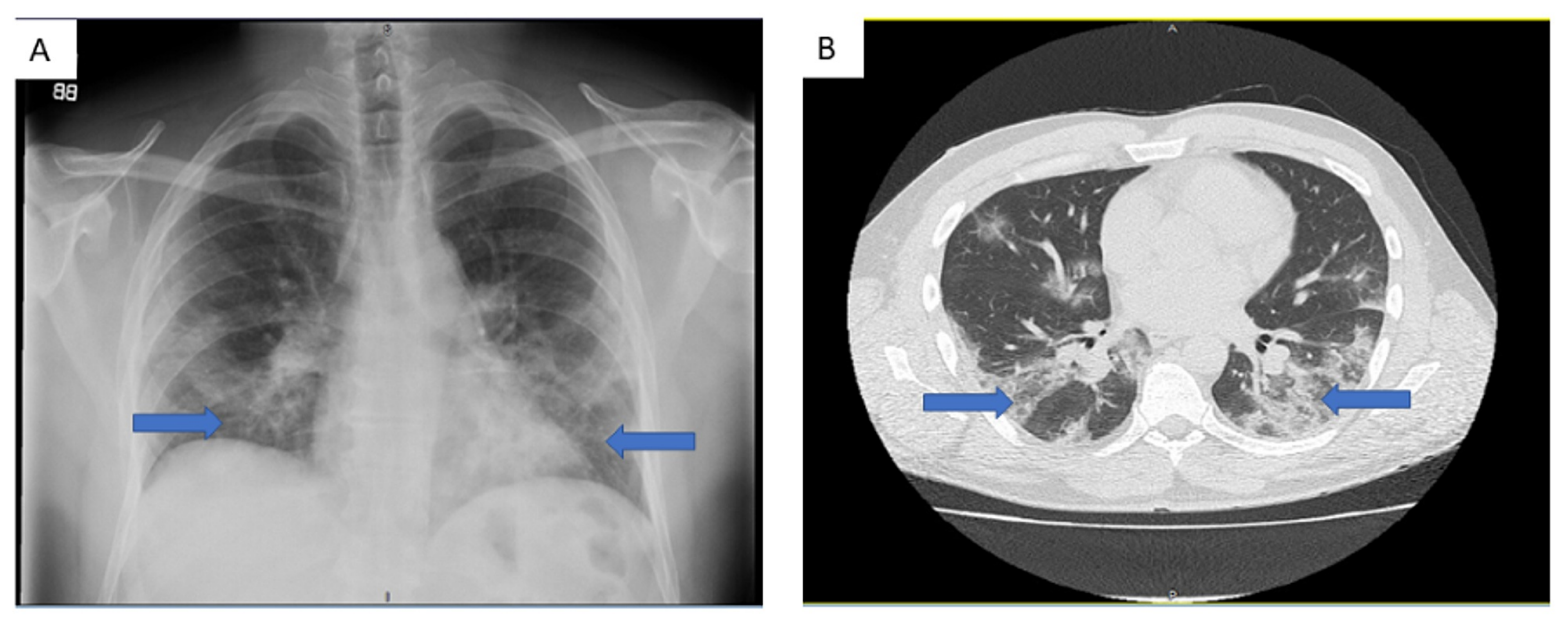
Imaging findings (a) Chest X ray and (b) CT scan findings on admission showing bilateral ground glass pulmonary opacities (blue arrows).
